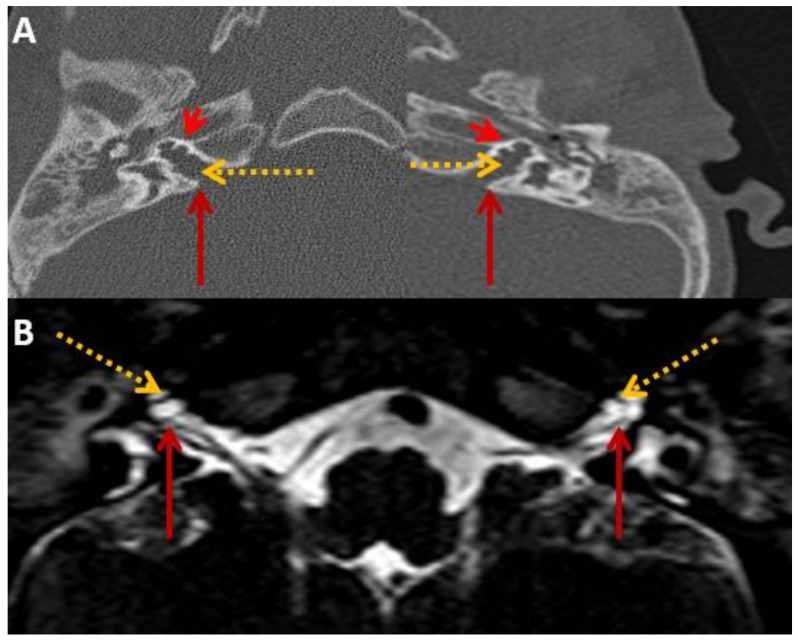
SB—HRCT and MRI. HRCT (A) and T2 Drive MRI (B) in sibling B showing bilateral bulbous dilatation involving the fundus of the internal auditory canal (maroon arrow) and bilateral incomplete separation of the basal turn of the cochlea (red arrowhead) from the fundus of the internal acoustic canal (dotted yellow arrow) classical of X-linked gusher disorder and similar to SA in Figure 2.
